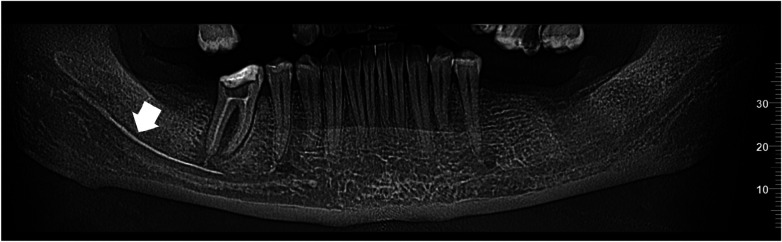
Panoramic CBCT scan showed hyperdense material emanating apically from tooth 46 extending along the mandibular canal.
